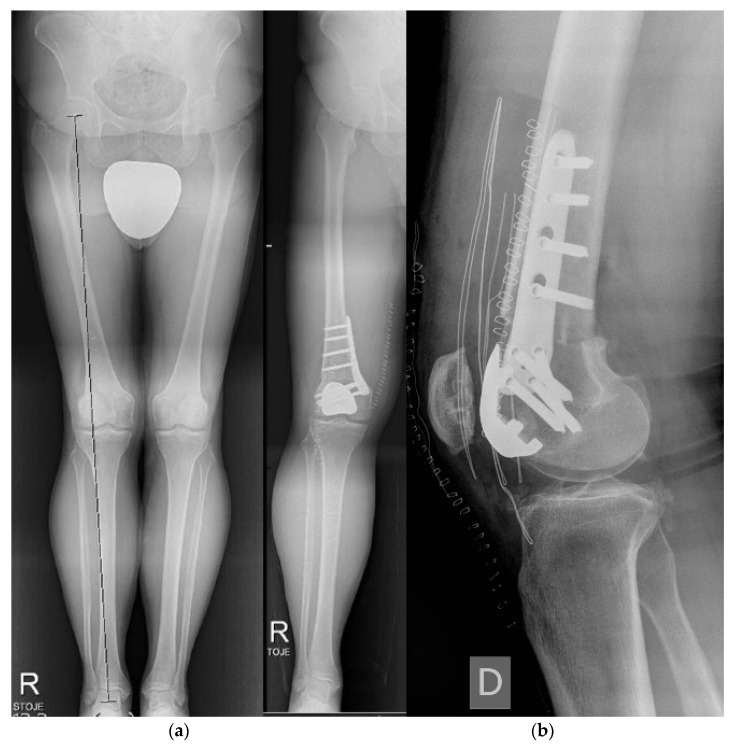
Patellofemoral arthroplasty. (a) preoperative X-ray of isolated patellofemoral osteoarthritis caused by torsional limb deformity; (b) postoperative X-ray after patellofemoral arthroplasty with concomitant distal femoral osteotomy (DFO) for limb deformity correction.
